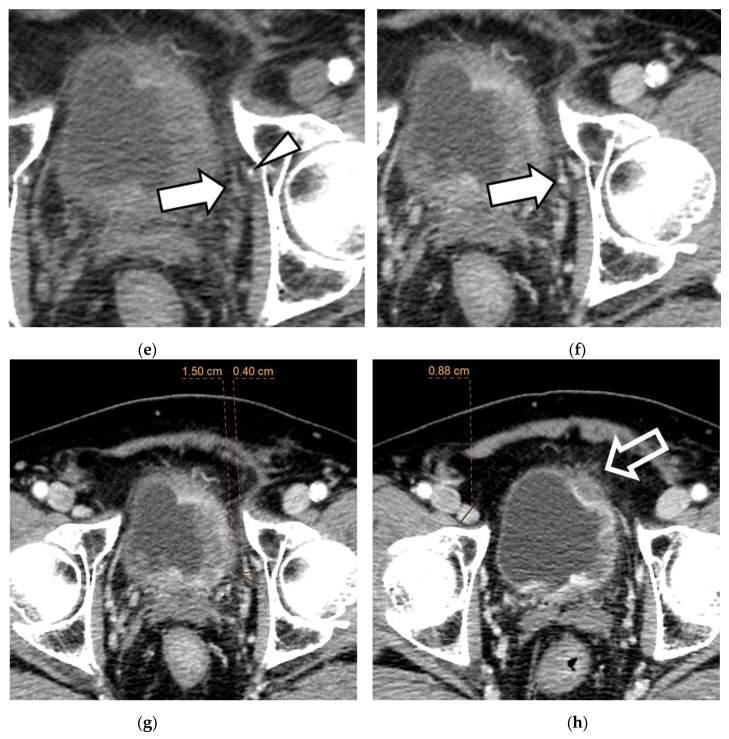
Bladder cancer, stage pT3b pN3. (a–h): Axial images from CT urography in the basal (a), arterial (b,e), venous (c,f,g,h), and urographic phase (d). In (a–d), the primary tumor (T) is highlighted by the letter “T”. (e,f): Assessment of the perivesical veins. The white arrow points to a perivesical vein (significant enhancement in (f), not in (e)), while the arrowhead points to an arterial vessel (e): the perivesical venous vessel was selected for measurement (white arrow). In g, the perivenous vessel shows a diameter of 4 mm and is within the distance of 15 mm from the bladder wall where the primary tumor is located. In a cranial image (h), a right iliac LN was measured (short axis of 8.8 mm), while the empty arrow points to perivesical solid tissue with perivesical fat stranding. In this case, the lymphovascular invasion (LVI) was also demonstrated at pathology.
